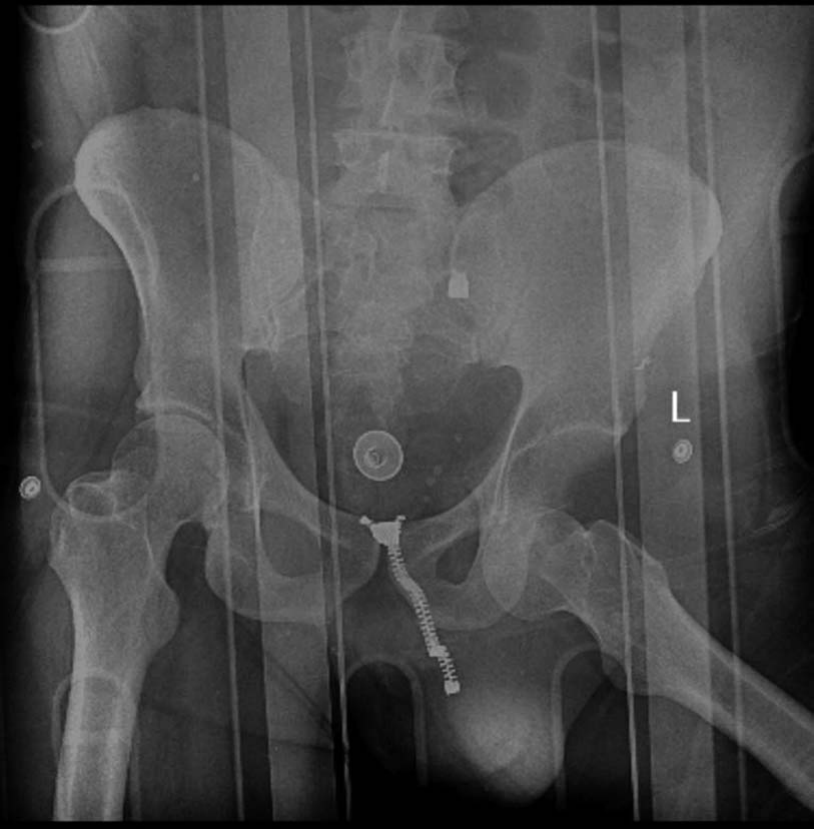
An anterior-posterior pelvic view X-ray. An inferior hip dislocation is seen in the left hip. No sign of fracture is seen.
